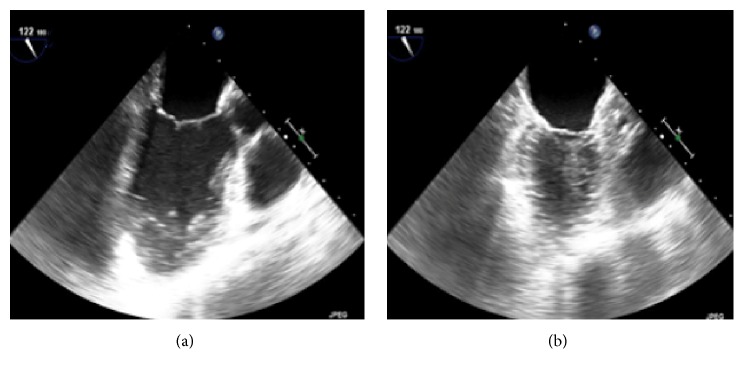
Transesophageal echocardiogram while off pentobarbital infusion, left ventricle ejection fraction of 50%: (a) end diastole; (b) end systole.
